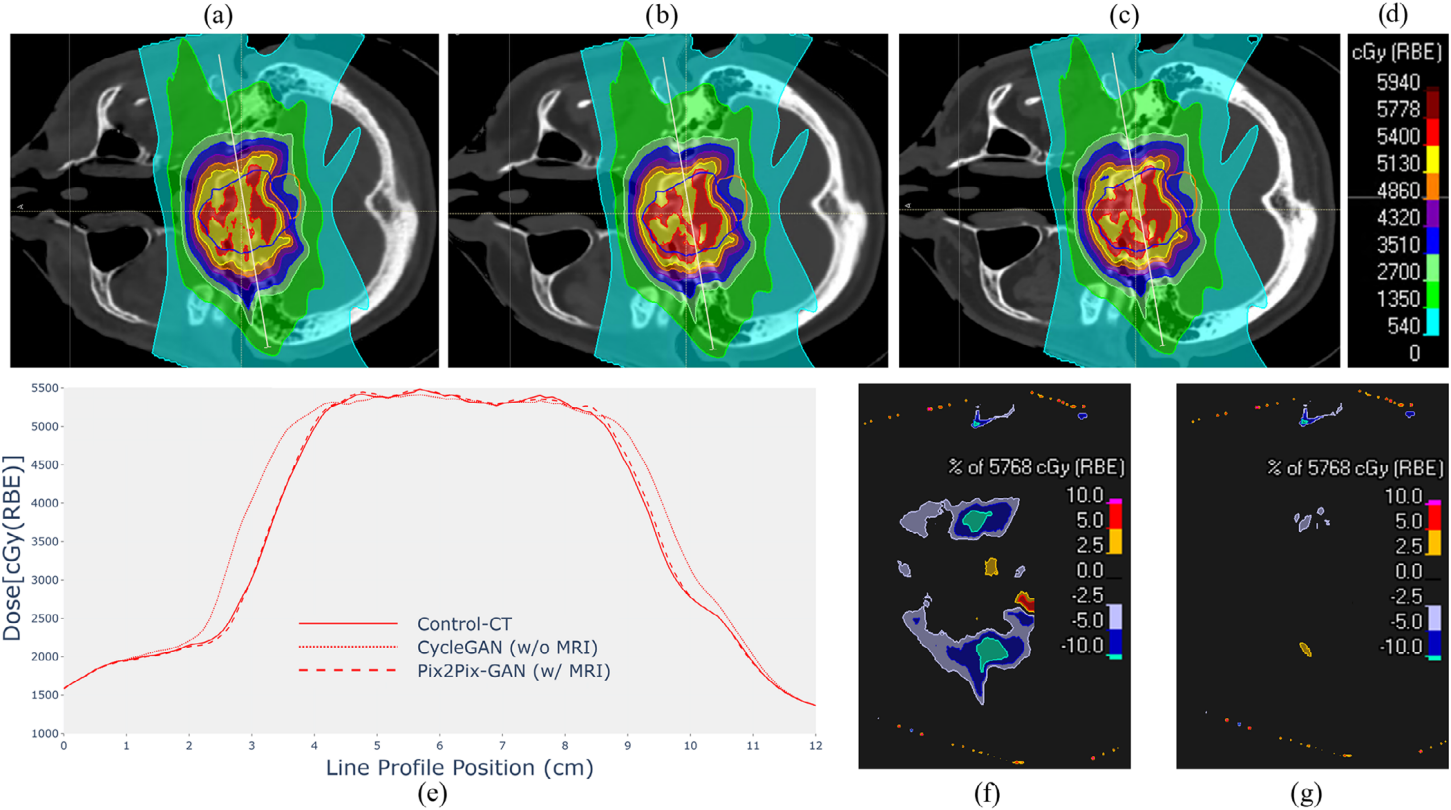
Dose distribution is shown on a specific slice for not MR‐based CycleGAN (a), MR‐based Pix2Pix‐GAN (b), and Control CT (c), with the color legend (d) of the dose values. Yellow line profiles showcased in those three top figures are compared in detail in the bottom chart (e). The map of dose differences compared to the Control CT is displayed for the non‐MR‐based model (f) and the MR‐based model (g). CT, computed tomography; CycleGAN, cycle‐consistent generative adversarial network; GAN, generative adversarial network.
